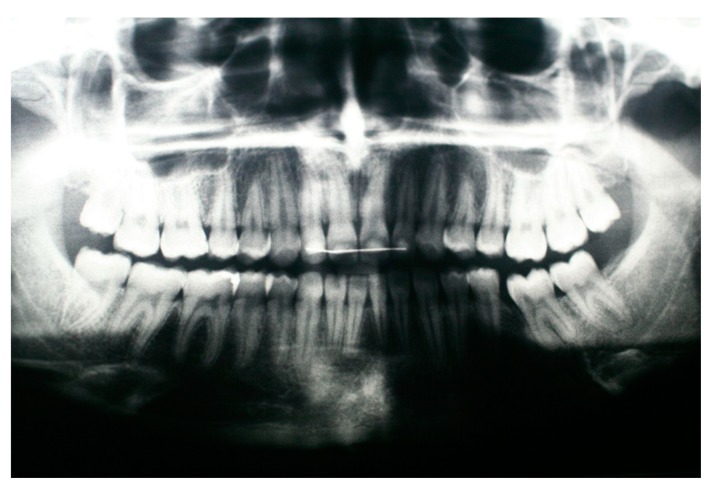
Panoramic radiograph 14 months after the debonding of the fixed orthodontic appliances and 32 months after the marsupialization of the dentigerous cyst.
